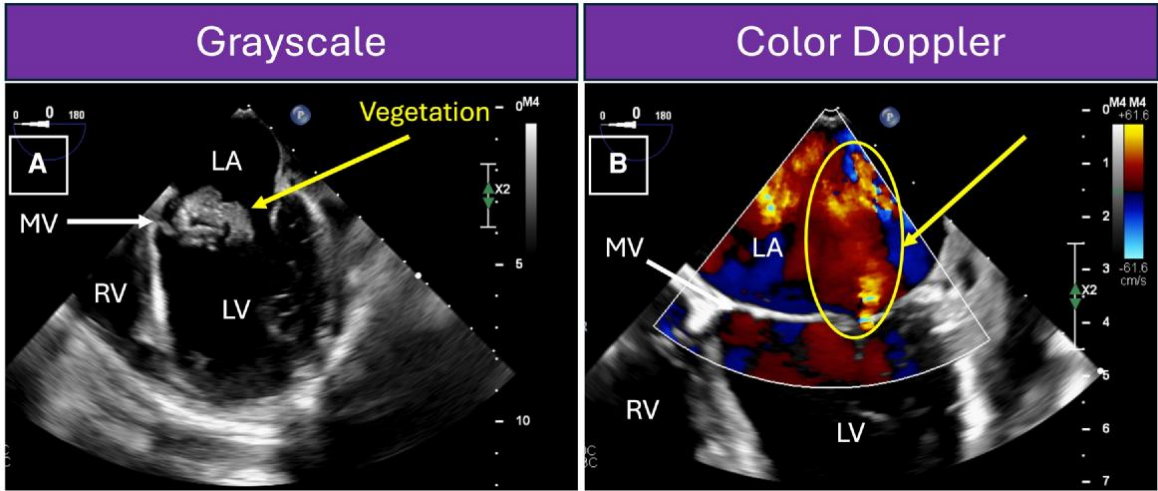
Case 1 (Mitral Valve Vegetation and Mitral Regurgitation): intraoperative transoesophageal echocardiogram (TEE) with midoesophageal two-chamber view at 0° in greyscale and colour Doppler. TEE better visualizes the base of the heart, including the left atrium and mitral valve, providing more precise details regarding the size and location of the mitral valve vegetation. When colour Doppler is placed over the mitral valve (*Panel B*), a red jet is seen in the left atrium, representing blood that is moving towards the probe during systole when the mitral valve closed. This represents mitral regurgitation. This figure corresponds to Supplementary material online, *Videos S3* and *S4*. LA, left atrium; LV, left ventricle; MR, mitral regurgitation; MV, mitral valve; RV, right ventricle.
